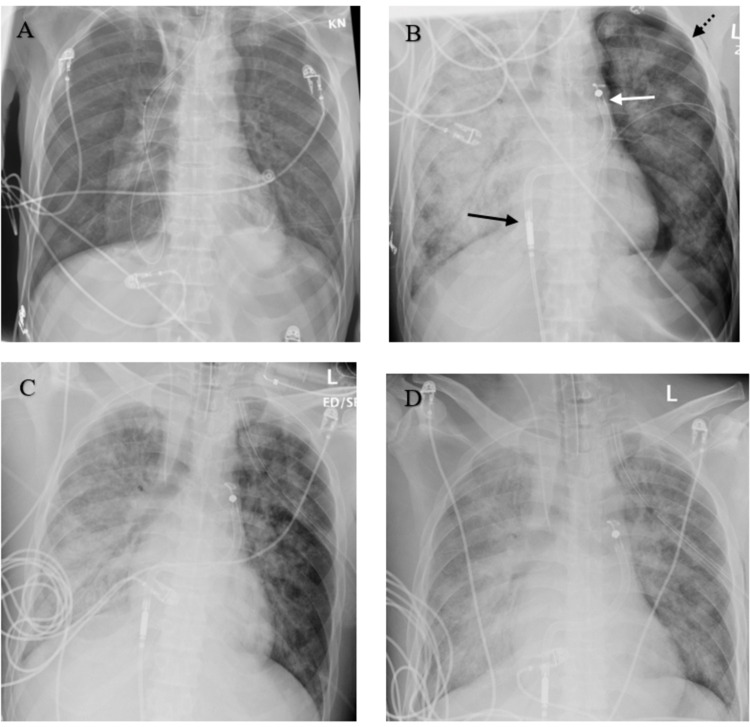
Serial chest X-rays. A. Day 1 of ICU stay ET tube in position. NG tube courses into the right lung. B. Day 3 (am): Right IJ approach central venous catheter with the tip projecting over the SVC. Significant increase in diffuse airspace opacification involving the entire right lung. Impella device visible (black arrow: Inlet area, white arrow: outlet area). Left-sided pneumothorax seen (black arrow with dotted line). C. Day 3 (pm): Interval placement of a left chest tube with the moderate volume left pneumothorax. No pneumothorax appreciated. D. Day 7: No significant interval change with near-complete opacification of the right lung and fluffy opacities throughout the left lung consistent with severe pulmonary edema. Small right pleural effusion.
